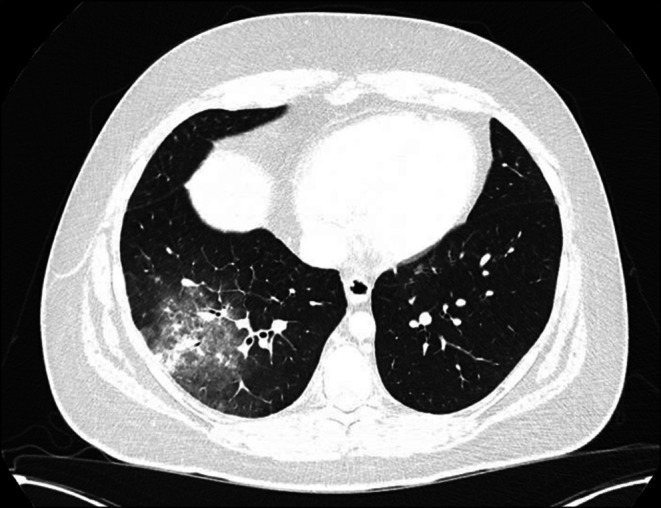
Transverse CT image of the thorax. Significant right lower lobe consolidation with ground‐glass opacification and interlobular septal thickening is visible.
